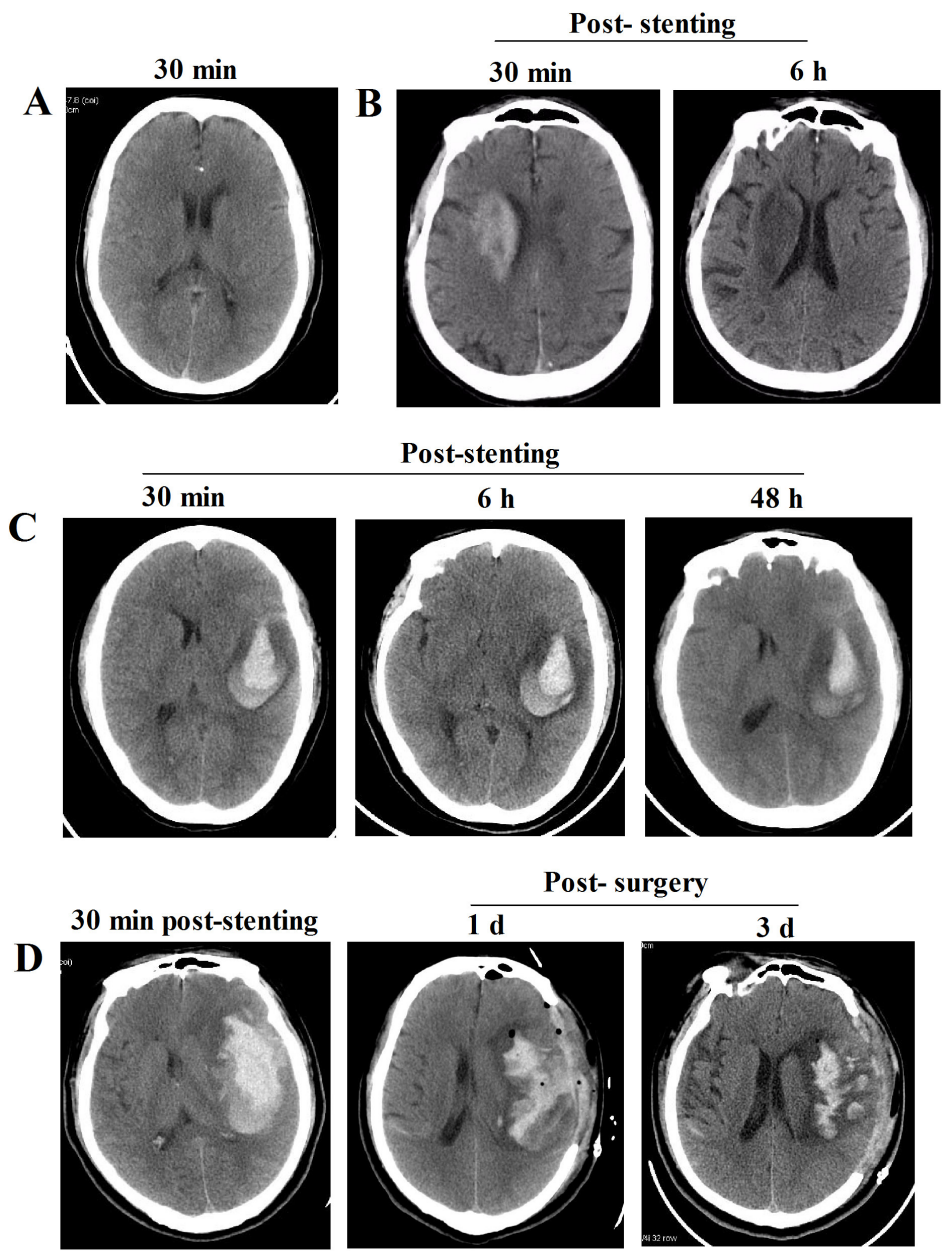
Representative images of the CT features in three Types after intracranial artery stenting (A) Representative CT image of patients in Type I. (B) Representative CT images of patients in Type II show that high-density shadows existed at 30 min after stenting were gradually disappeared within the next 24h. (C) Representative CT images of patients in Type IIIa show that high-density shadows occured at 30 min, which was not expanded at 6 h post-stenting, while the high-density shadow was shrank at 48 h after stenting. (D) Representative CT images of patients in Type IIIb show that high-density shadows existed at 30 min after stenting were gradually aggravated with obvious mass effects within next 6 h, then immediately receiving surgical operation.
